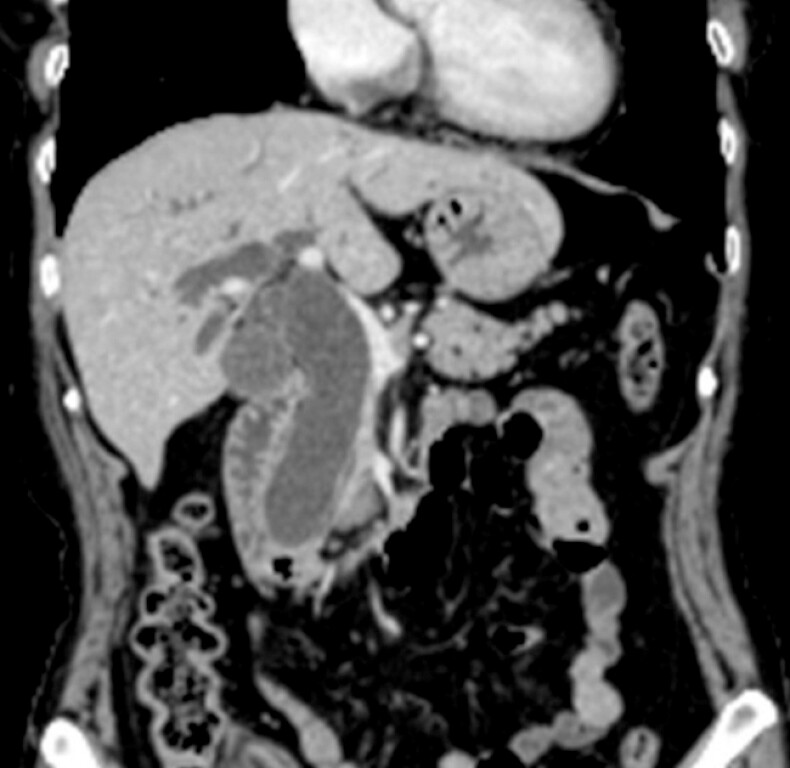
Contrast-enhanced computed tomography shows duodenal papillary carcinoma and dilation of the common bile duct.
